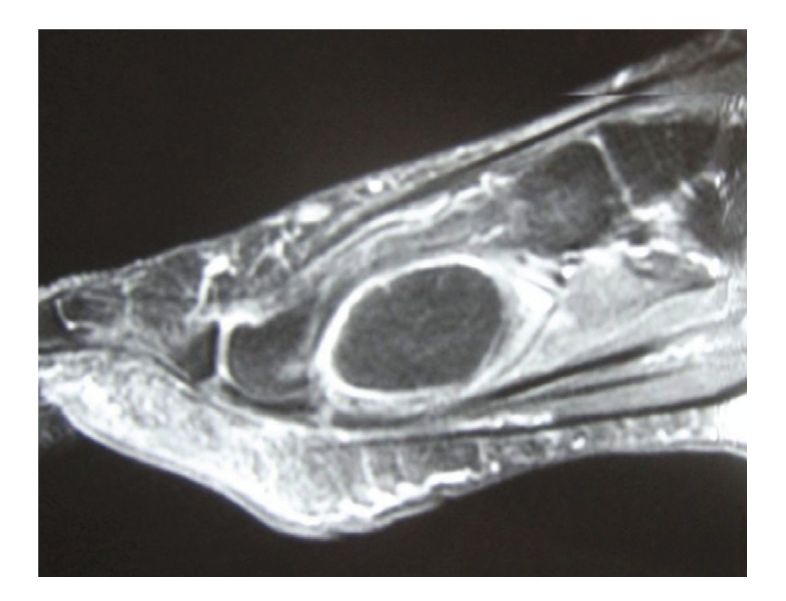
Sagittal gadolinium-enhanced MRI at admission.
